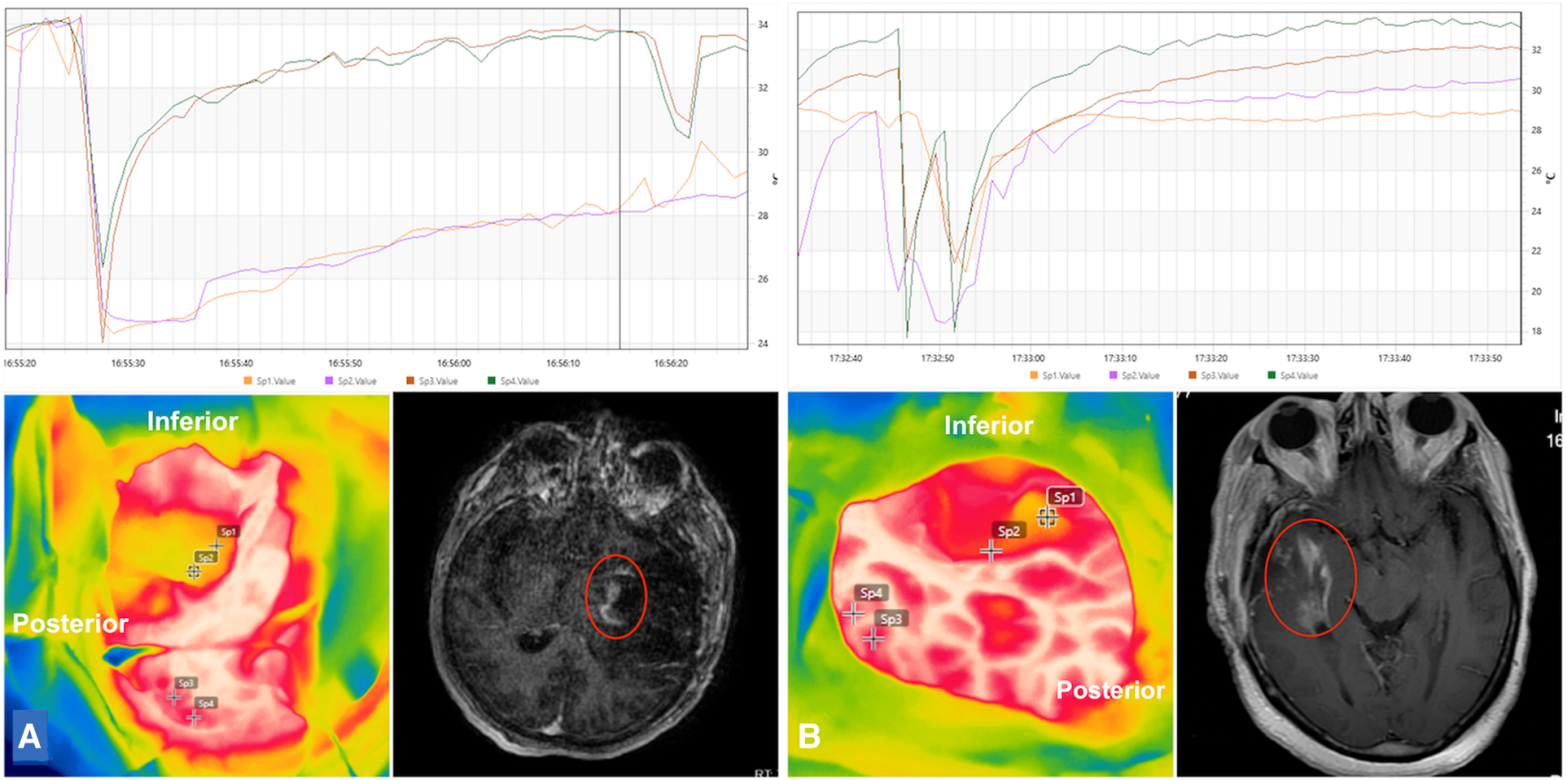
Intraoperative infrared thermographic imaging of the tumor beds of two temporal glioblastomas (A,B; lower left). Suspected areas of delayed temperature recovery curves were marked and correlated to postoperative imaging. Residual tumoral areas thermographic profiles demonstrated similar thermal recovery curves of tumoral areas obtained before tumor resection, namely steady and slow temperature recovery and lower final temperature (orange and purple lines in graphs) in comparison to the healthy cortex (dark orange and green lines in graphs) (A,B; upper row). Postoperative gadolinium-enhanced T1-weighted images demonstrated residual tumors at the tumor beds (A,B; lower right).
